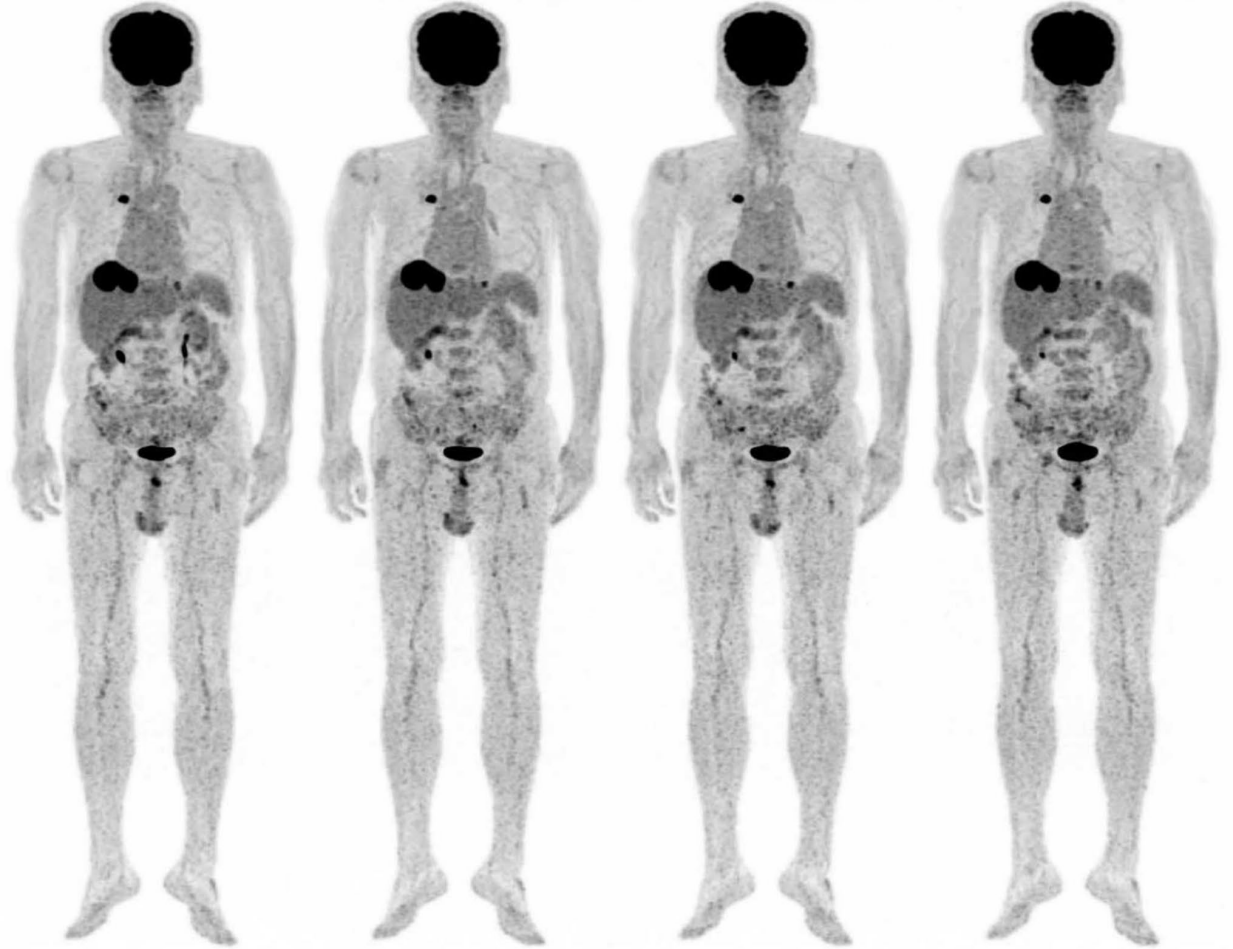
Adapted from Tamaki et al. 2023 [22]. Sequential whole-body FDG-PET scans (3 min each) performed about 60 min post-FDG injection in a patient with lung cancer and pulmonary metastatic lesions. The scans demonstrated strong and sustained uptake in both the primary lung cancer and liver metastases, while uptake changes due to motion were observed in the ureter and small intestine. This distinction helps in differentiating between pathological and non-pathological abdominal accumulations
